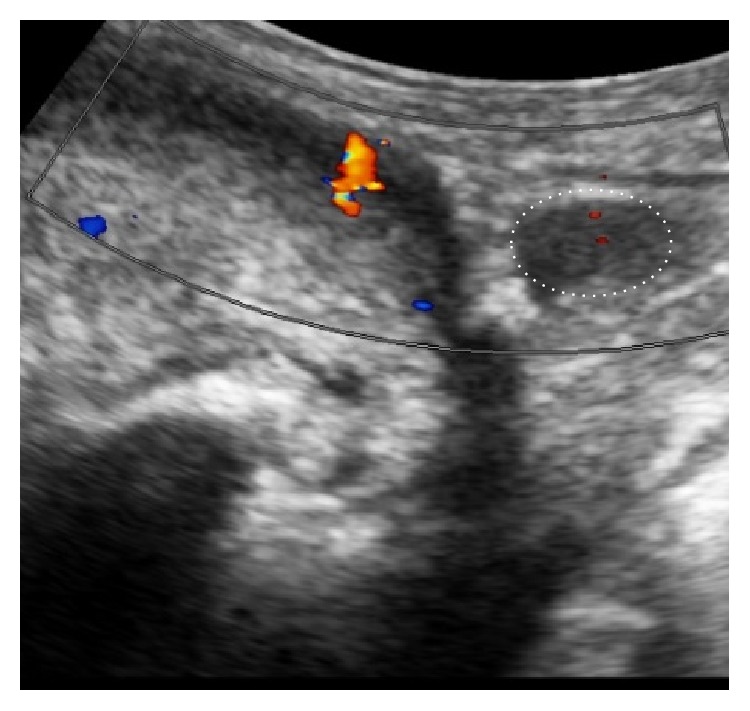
Duplex Doppler showing blood flow after sling placement. Blood flow within urethral bulb after sling placement is maintained. Note the healthy (>1 cm long) proximal portion of urethral bulb on the right side (white dotted ellipse).
